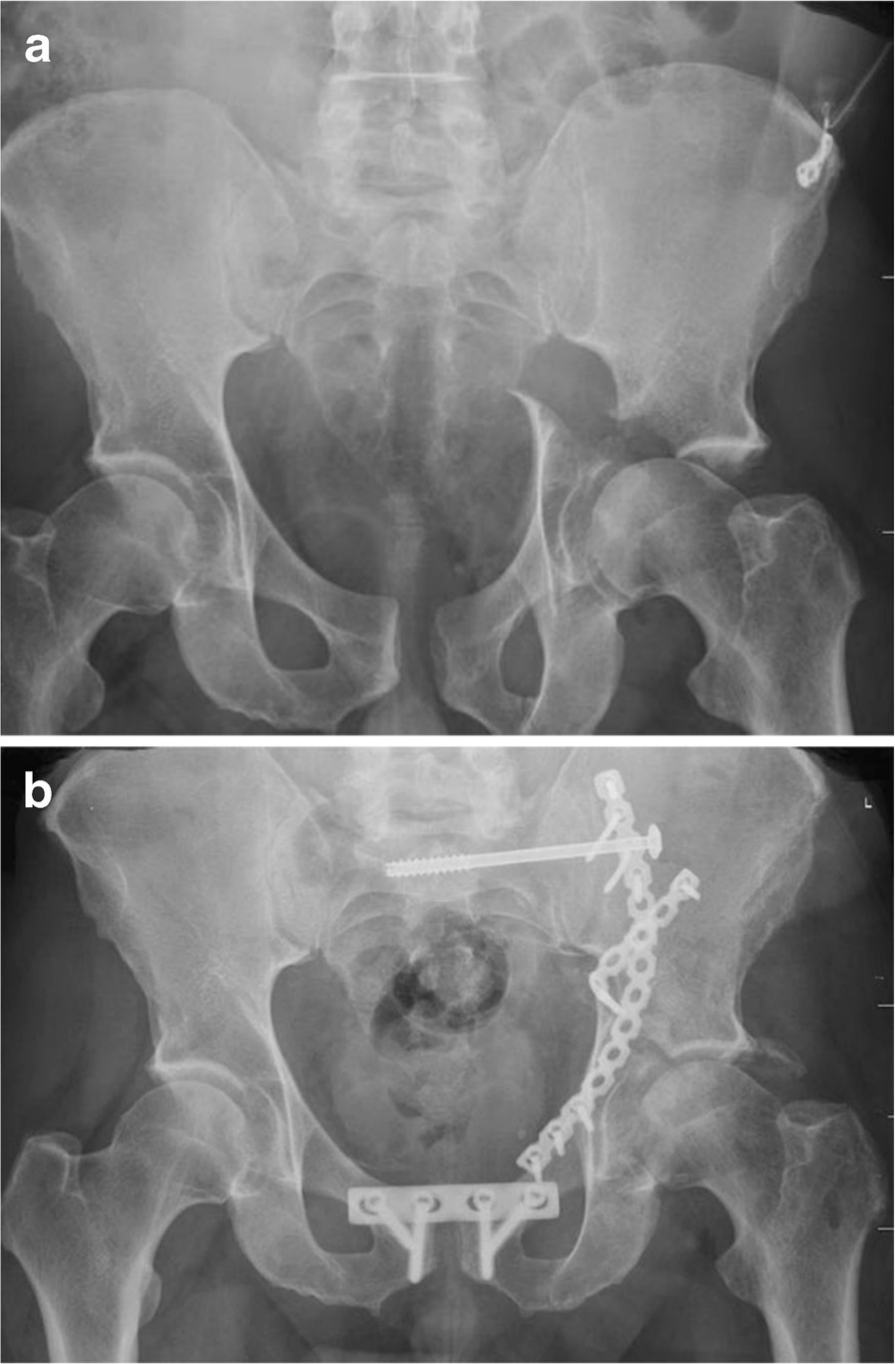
a) This is a x-ray of a 48 year old motor cyclist, who suffered an accident with a both column acetabular fracture accompanied by a pelvic ring injury. Due to a massive soft tissue involvement of the pelvis and prolonged wound healing, an isolated ilio-inguinal approach was applied to reduce and stabilize the fracture. Therefore a nonanatomical reduction had to be accepted. b) X-ray 4 months after surgery. The combination of great initial displacement and nonanatomical reduction led to a rapid joint degeneration with poor clinical function.
